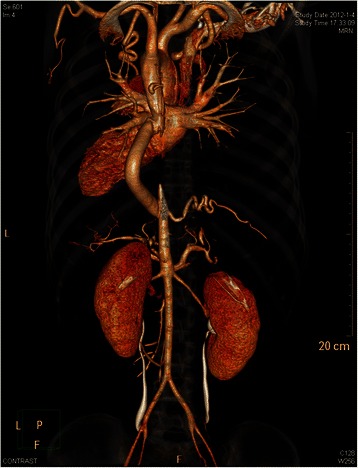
Postoperative chest computed tomography and three-dimensional reconstruction images demonstrating good graft patency
